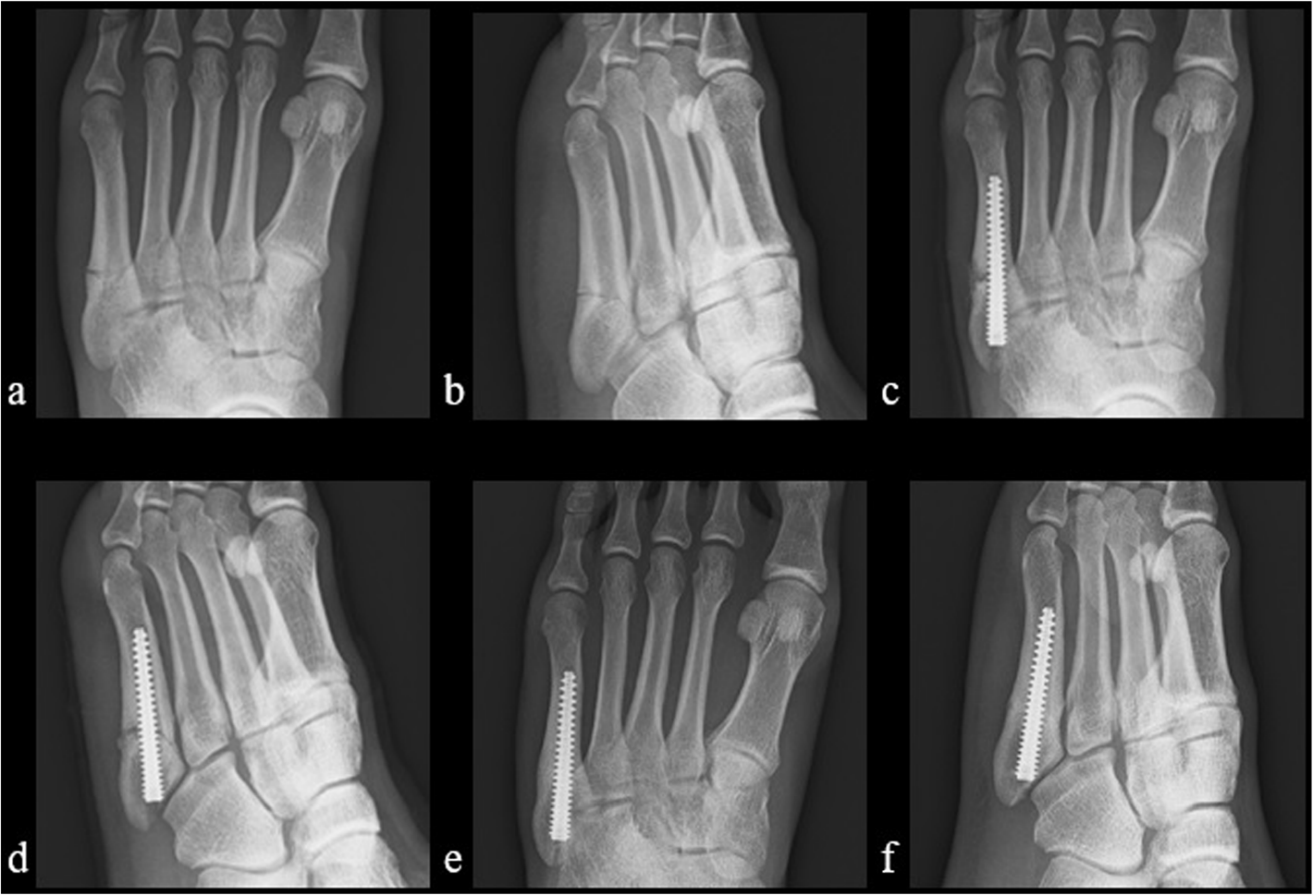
Case presentation. A 17-year-old patient with LIPUS treatment after intramedullary screw fixation. a and b. Radiographs showed proximal metatarsal stress fracture preoperatively (a. anterior-posterior and b. oblique view). c and d. Radiographs in the immediate postoperative period (c. anterior-posterior and d. oblique view). e and f. The radiographs obtained 11 weeks postoperatively showed bone union (e. anterior-posterior and f. oblique view)
